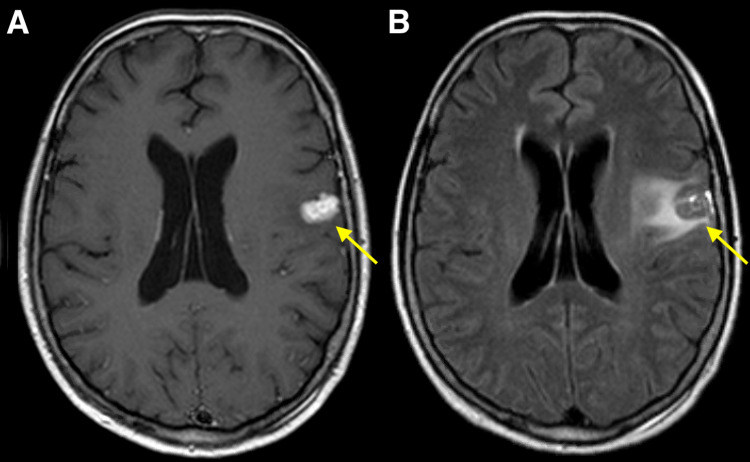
Gamma Knife stereotactic radiosurgery planning MRI. Axial contrast-enhanced T1-weighted (A) and T2-weighted FLAIR brain MRI (B) at the time of Gamma Knife stereotactic radiosurgery demonstrating enhancing left frontal metastasis measuring 1.6 cm with surrounding edema. FLAIR: Fluid-attenuated inversion recovery
